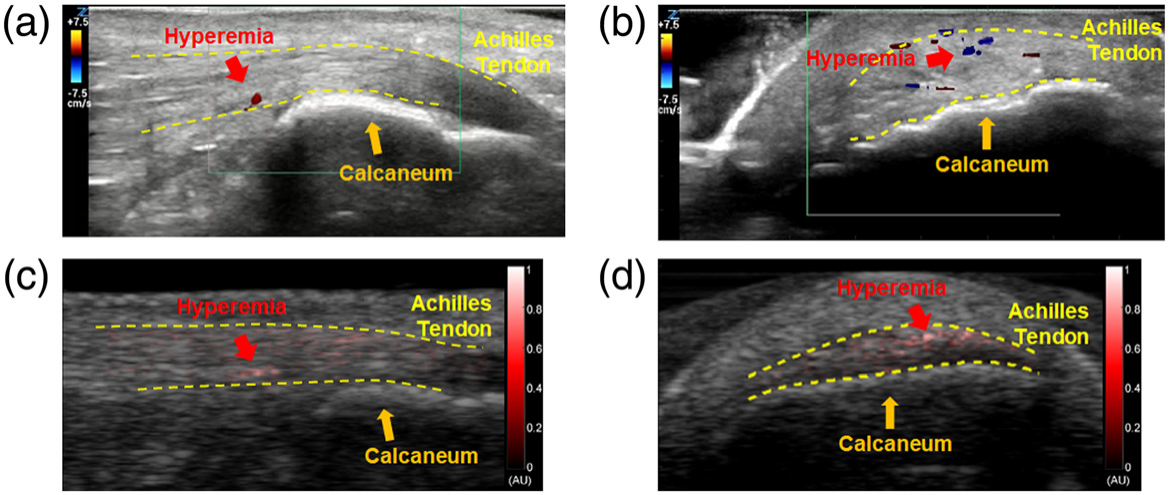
US Doppler and PA imaging of an Achilles tendon (margins outlined by dotted line) of an SpA patient. (a) and (b) US Doppler images of the patient’s Achilles tendon when scanned along the long and the short axes of the tendon, respectively. (c) and (d) PA images of the patient’s Achilles tendon when scanned along the long and the short axes of the tendon, respectively. The pseudocolor PA images were superimposed on the gray-scale B-mode US images.
